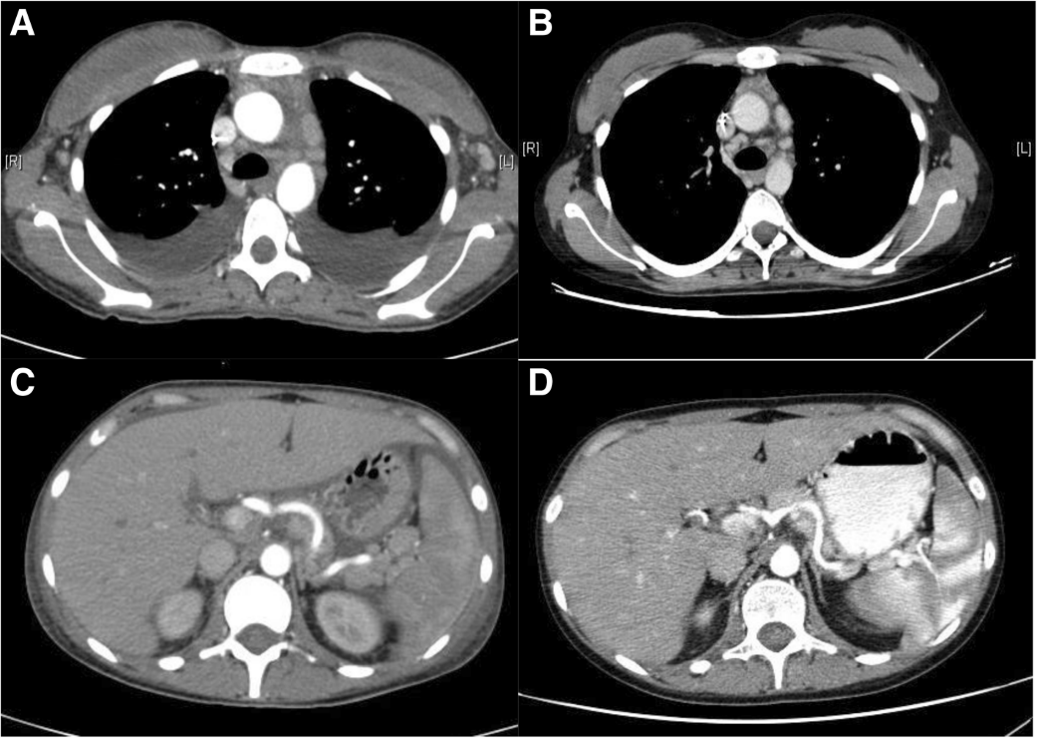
CT images. (A, B) Contrast enhanced thoracic computed tomography (CT) before (A) and after (B) six months of chemotherapy, showing resolution of pleural effusion and the shrinking of axillar and mediastinal lymphnodes. (C, D) Contrast enhanced abdominal CT before (C) and after (D) six months of chemotherapy, showing the reduction of hepato-splenomegaly and celiac and perisplenic lymphadenopathy.
